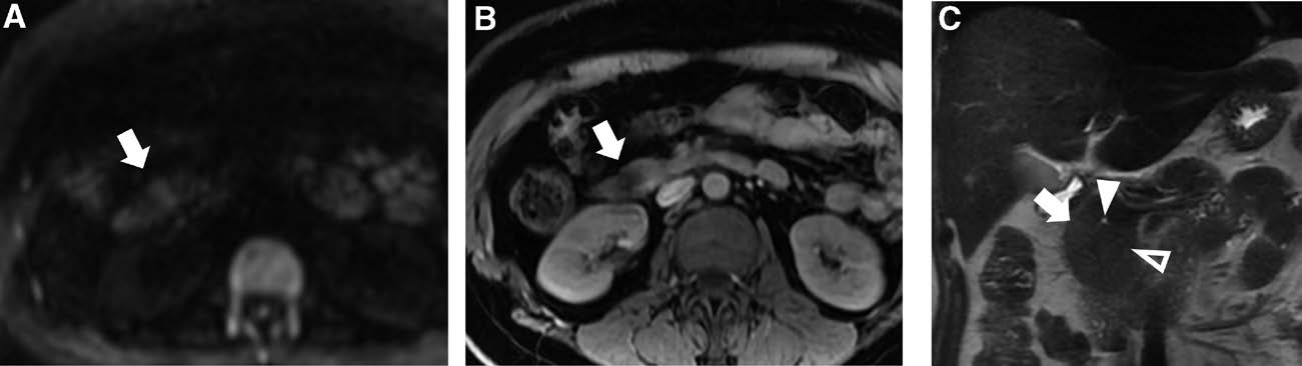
Pre-operative contrast-enhanced abdominal MRI. (a) Diffusion-weighted imaging (DWI) revealed a high signal intensity (SI) nodule in the pancreatic head (white arrow). (b) Fat-saturated T1-weighted imaging (fs-T1WI) revealed the tumor as a low-SI nodule (white arrow). (c) On coronal T2-weighted imaging (T2WI), the tumor was slightly hyper-intense compared to the pancreas, which is barely visible (white arrow). The white arrowhead and the hollow arrowhead indicates the duct of Santorini and the duct of Wirsung, respectively. MRI = magnetic resonance imaging.
